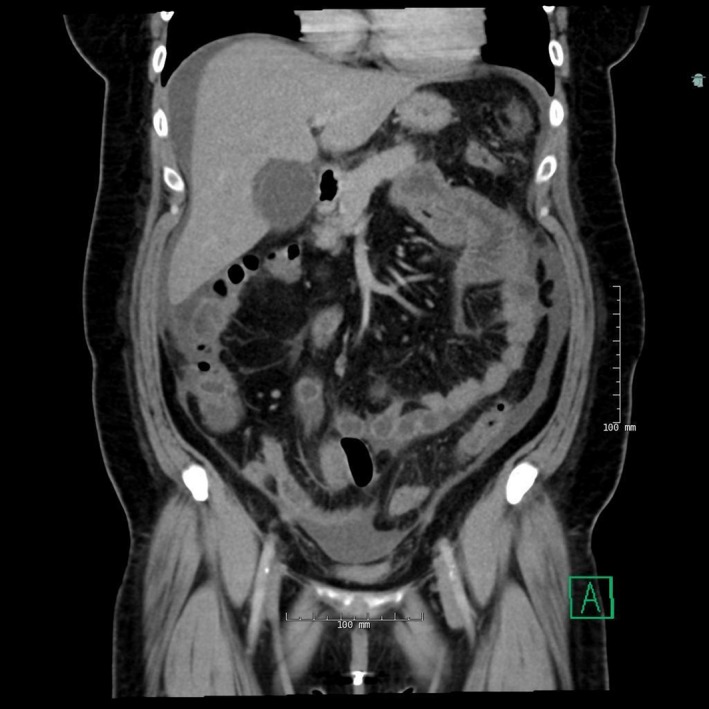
CT abdomen and pelvis coronal view demonstrating minimal ascites around liver
